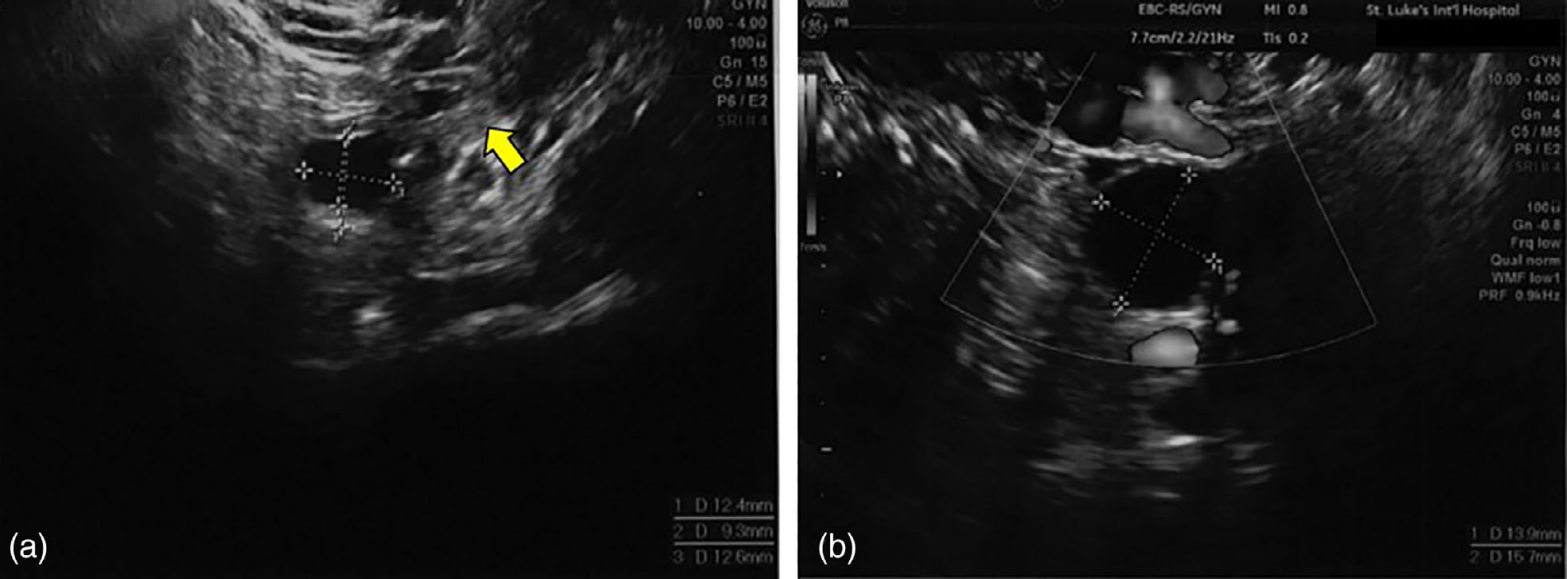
Follicular development findings by ultrasound. A follicle is seen outside the remaining left ovary (yellow arrow) (a); it continues to develop till the next day (b)
